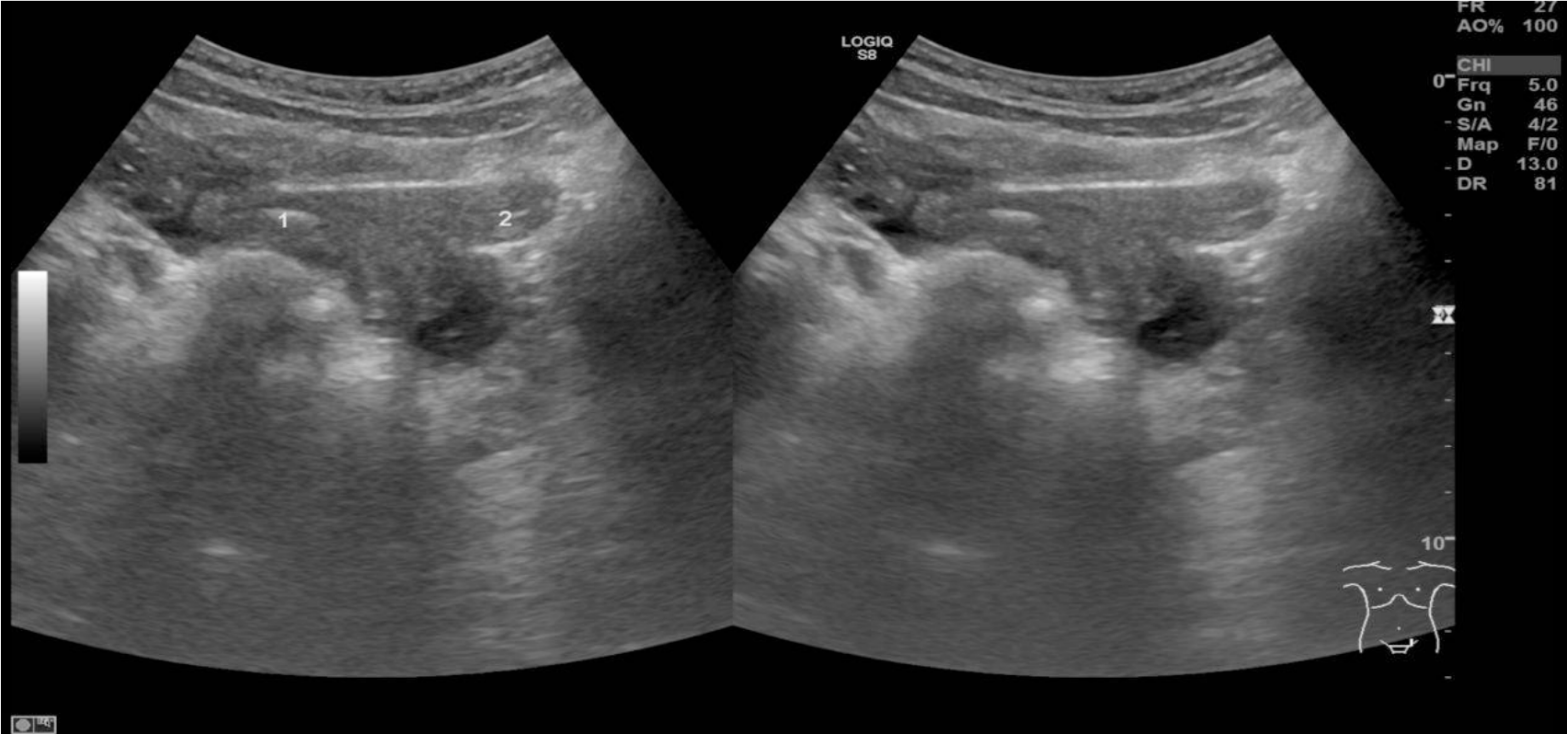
Ultrasound images of double corpuses of uterus with two separate linings of endometrium.
